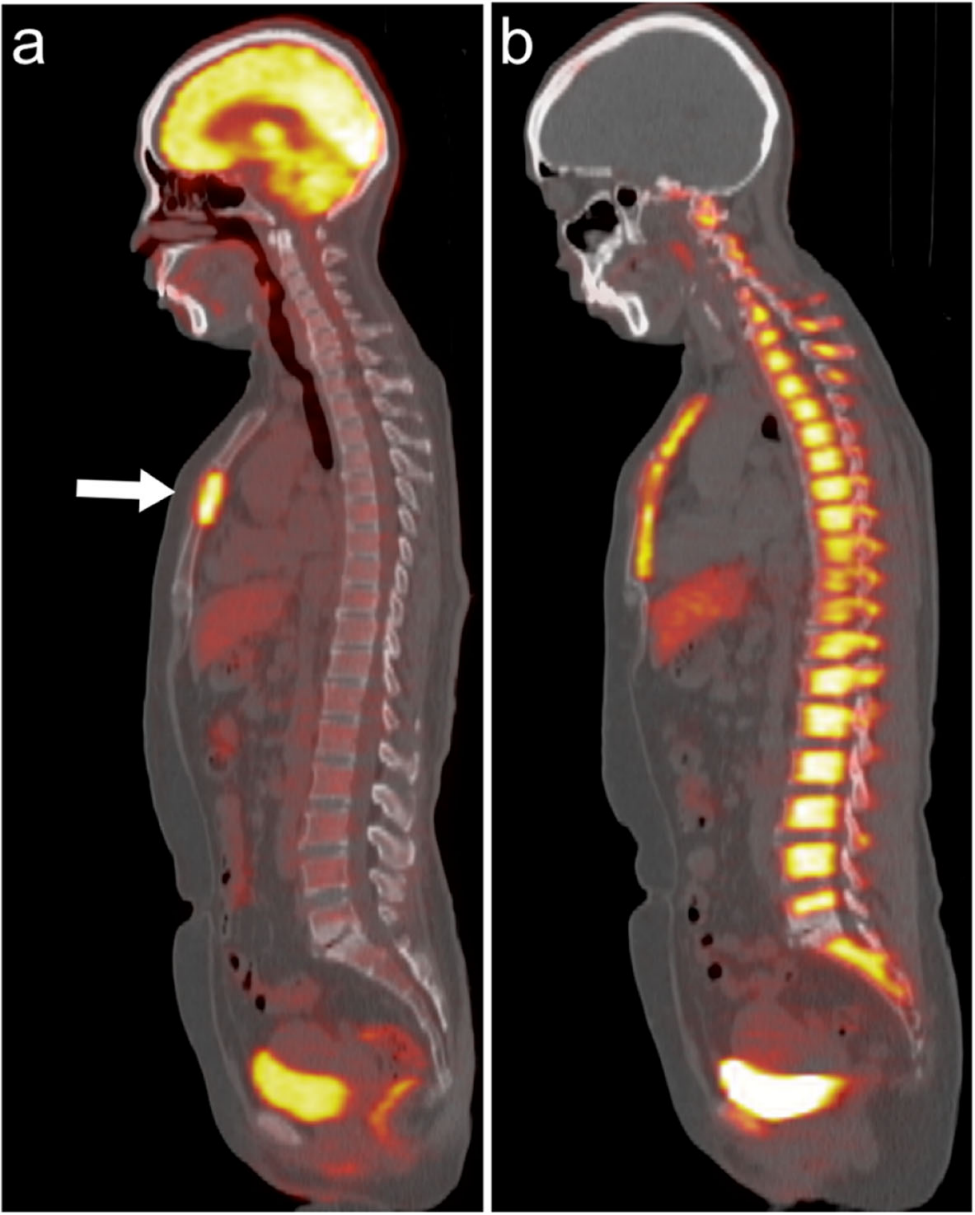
A case with metastatic breast cancer at the sternum. (A) ^18^F-FDG-PET, (B) ^18^F-FLT-PET. The lesion was clearly demarcated in ^18^F-FDG-PET. In ^18^F-FLT-PET, the margin of the lesion could not be well defined due to physiological ^18^F-FLT uptake in the adjacent bone marrow
